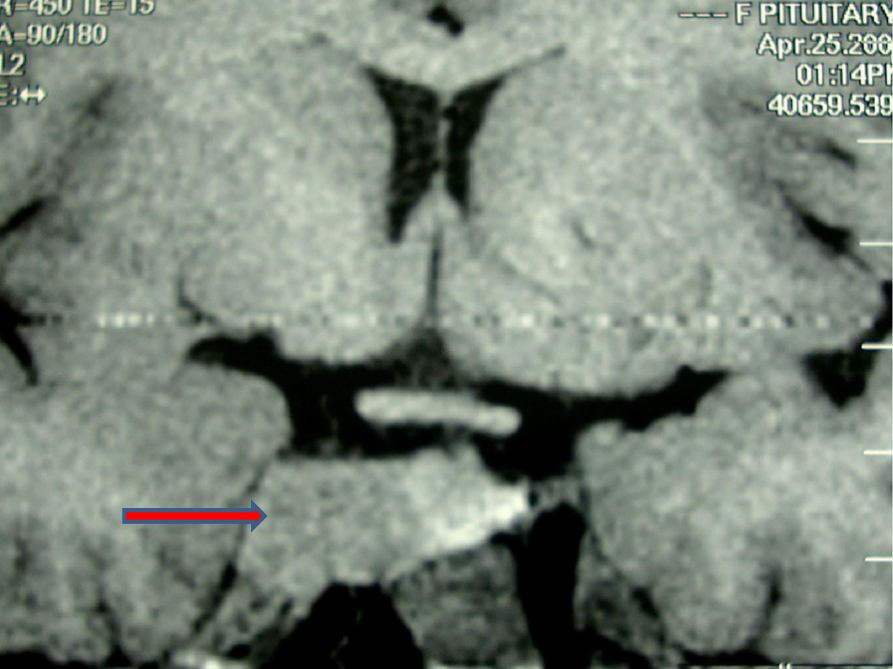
MRI (T1 weighted image) showing a Pituitary Macroadenoma measuring 2.2 cm × 2 cm × 1.3 cm (marked with a red arrow), with minimal supra-sellar extension, involving the right cavernous sinus with encasement of internal carotid artery and extending into the optic canal abutting the optic chiasm superiorly.
